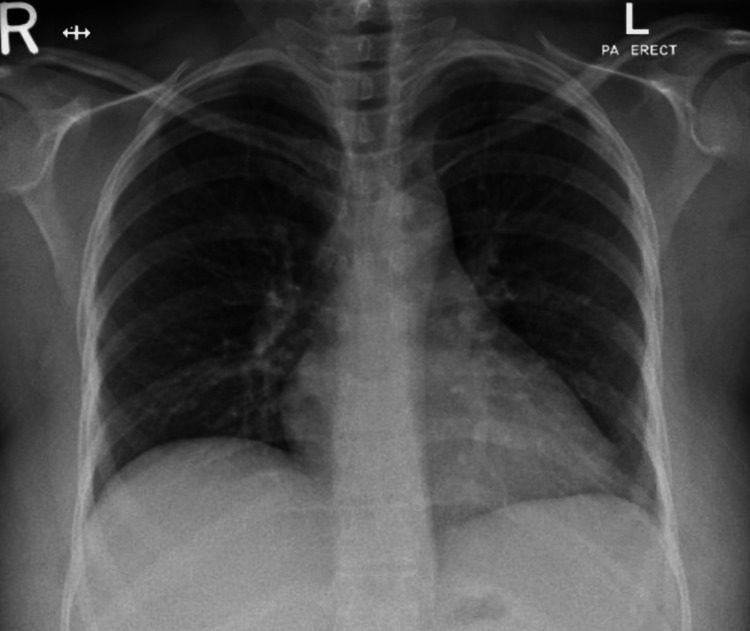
Chest X-ray after treatment showing a significant improvement of the widespread areas of airspace opacities in the bilateral lung zones
